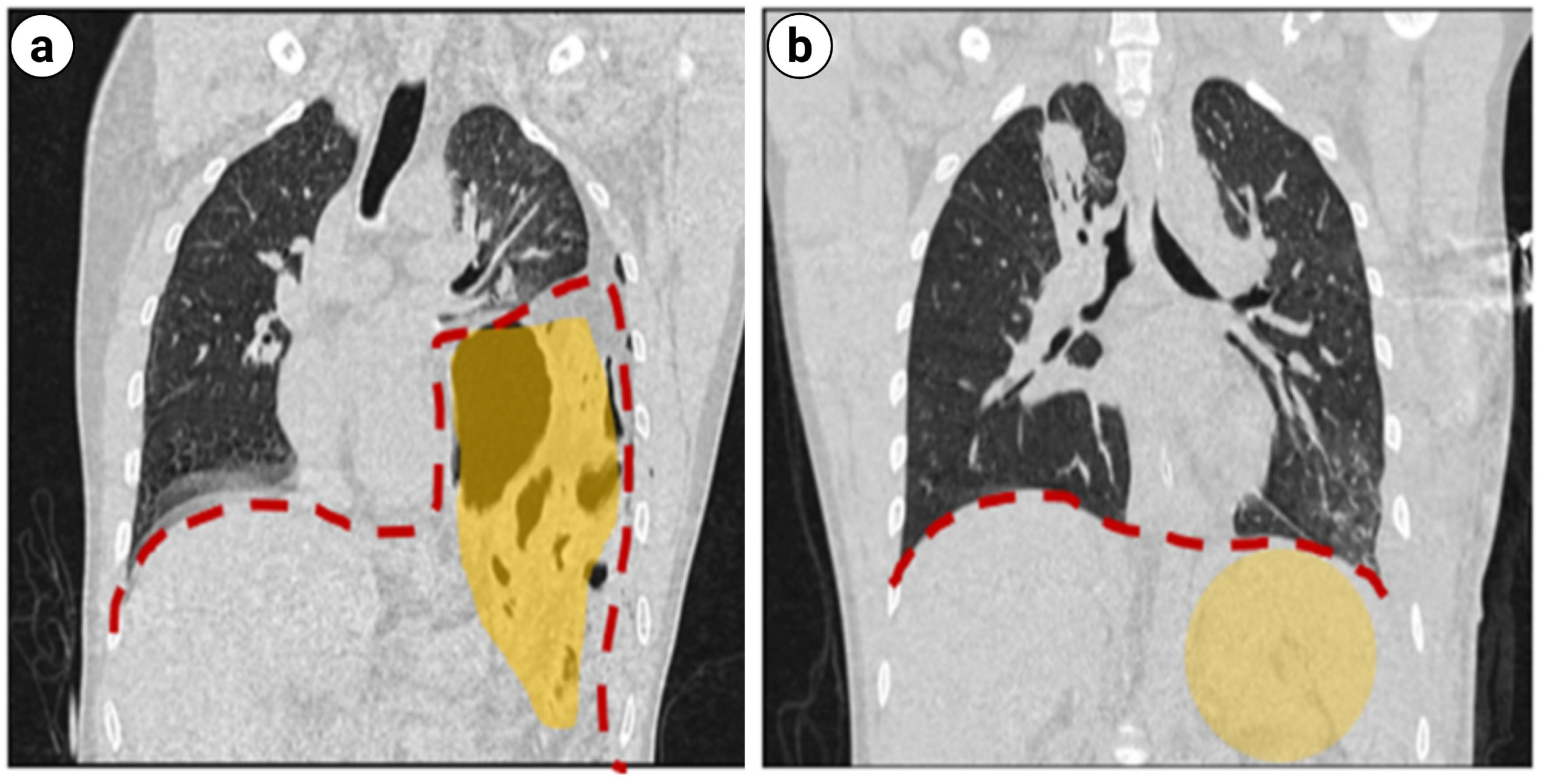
Coronal CT scan of the chest: (a) Preoperative traumatic diaphragmatic hernia reduction. (b) Postoperative traumatic diaphragmatic hernia reduction (within the yellow shaded area).
